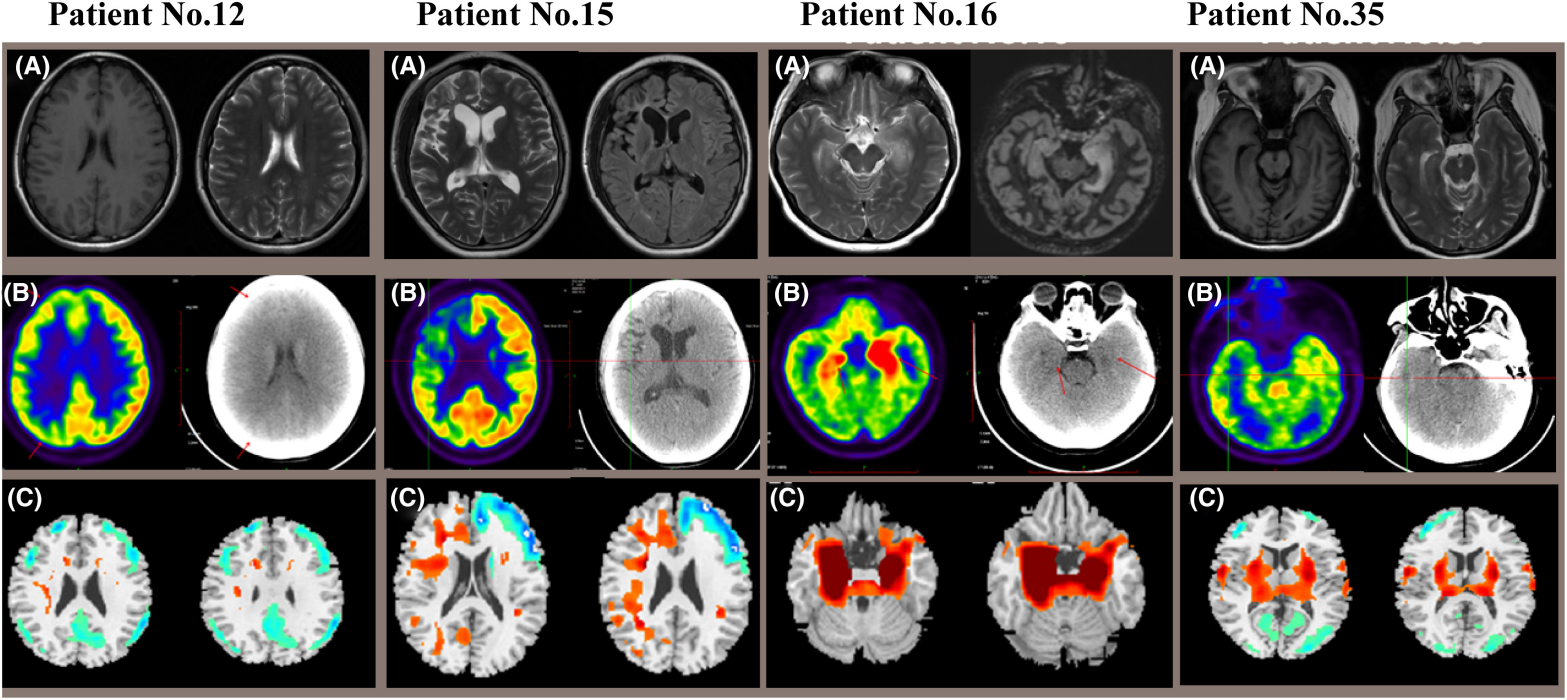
Corresponding MRI images, abnormal cerebral glucose metabolism patterns and ^18^F‐FDG PET/CT results based on SPM of 4 patients with AE. (A) MRI imaging, (B) cerebral glucose metabolism patterns, (C) ^18^F‐FDG PET/CT results based on SPM (*p* < 0.05, FWE corrected). The hypermetabolism regions were shown in orange‐red color, and hypometabolism regions were shown in blue‐green color. ^18^F‐FDG PET/CT, 2‐deoxy‐2‐^18^Ffluoro‐D‐glucose‐(^18^F‐FDG) positron emission tomography/computed tomography; AE, autoimmune encephalitis; FWE, family‐wise error; MRI, magnetic resonance imaging; SPM, Statistical Parametric Mapping.
